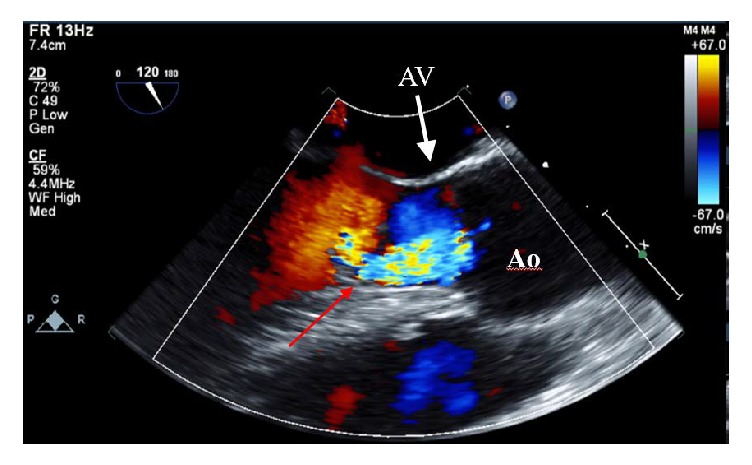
Transesophageal echocardiogram with color flow Doppler showing the aortic regurgitant jet (blue color) contacting the septal wall of the left ventricular outflow tract at the attachment site of the mass (red arrow). Ao = aorta and AV = aortic valve.
